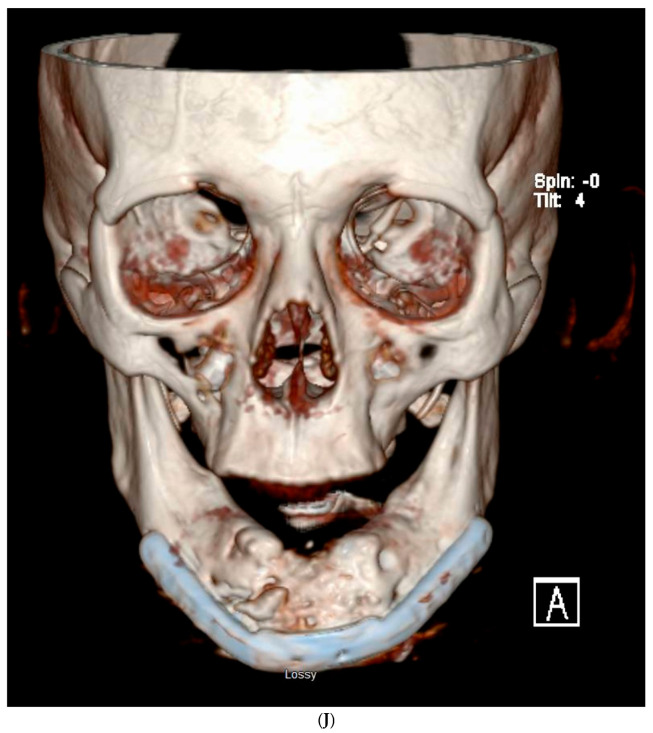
A 44-year-old female that was shot in the face that had original reconstruction plate removed secondary to intra-oral exposure and infection with reconstruction with autogenous bone graft and platelet-rich fibrin. (A) Initial CT scan demonstrating mandibular fracture. (B) Post-operative CT scan completed by another surgeon demonstrating continuity defect. (C) Planned removal of infected plate and bone. (D) Patient-specific reconstruction plate and titanium crib. (E) Intra-operative photograph demonstrating infected-plate removal. (F) Patient-specific reconstruction plate in place. (G) Autogenous bone graft in syringe and platelet-rich fibrin membranes. (H) Bone graft being placed into defect. (I) Bone graft in place with platelet-rich fibrin membranes over graft. (J) Post-operative CT scan demonstrating reconstruction of mandibular continuity defect.
